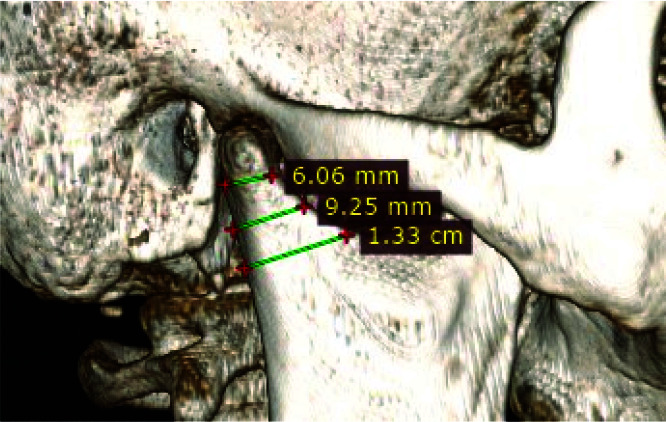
Dimensions of condylar neck region in computed tomography scan
